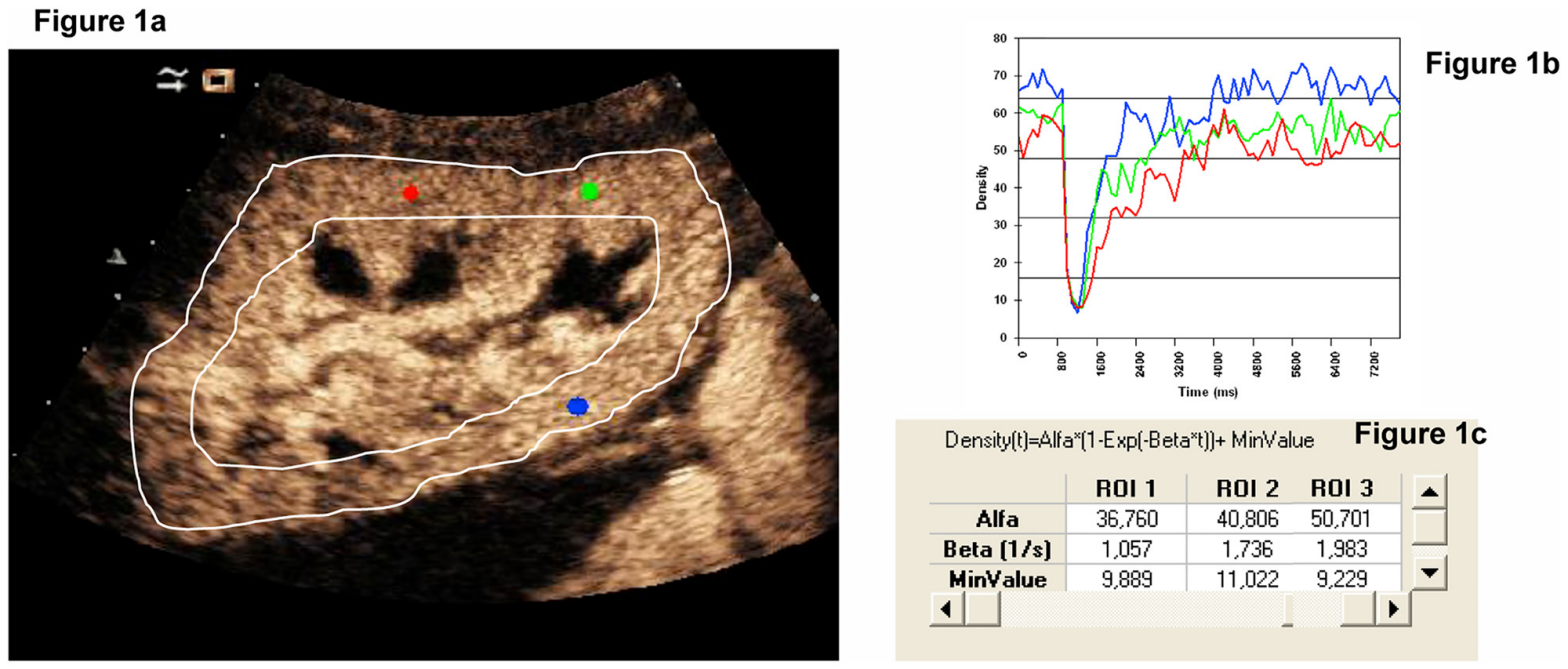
This figure shows renal graft perfusion by contrast media in a longitudinal view. Red, green and blue circles are regions of interest selected in the proximal and distal renal cortex. The white line identifies and isolates the renal cortex. Once uniform graft perfusion was achieved, microbubbles were destroyed with a high mechanic ultrasound pulse. The replenishment kinetics was recorded stored in order to perform time-intensity curves using a software tool (Fig 1b). Cortical capillary blood flow for each region of interest could be estimated (Fig 1c).
